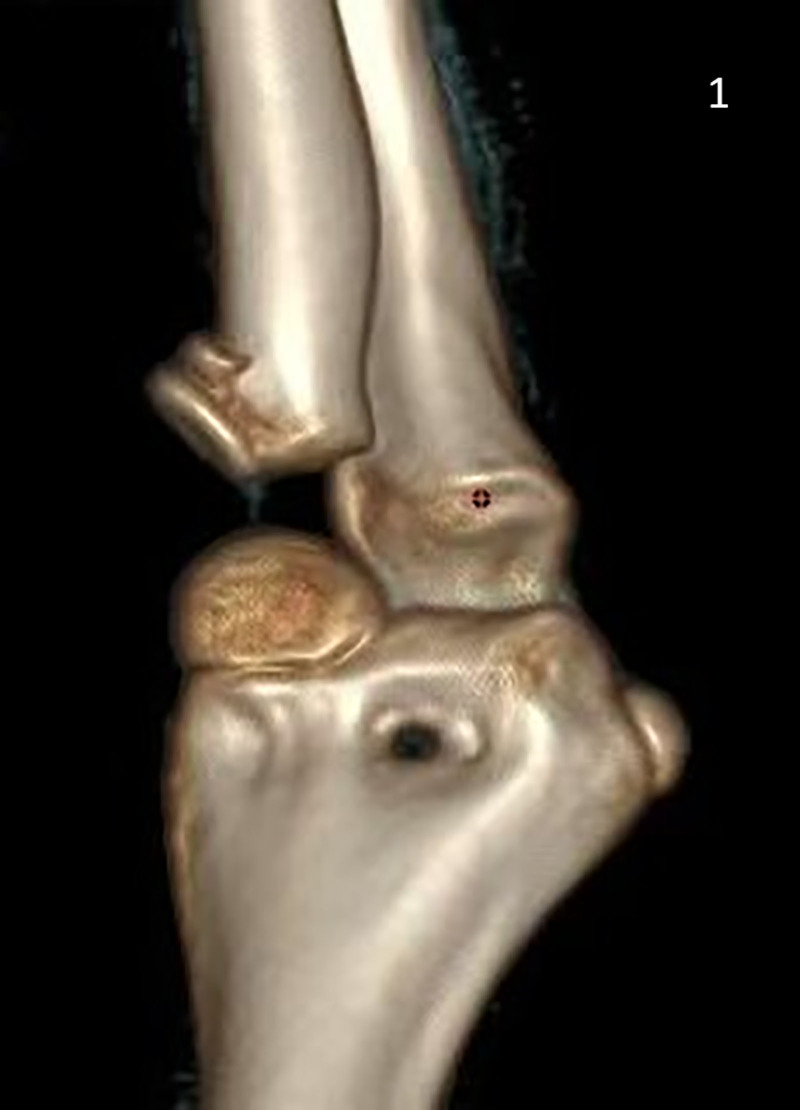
3D CT examination suggested “left radial head fracture.” CT = computed tomography.
